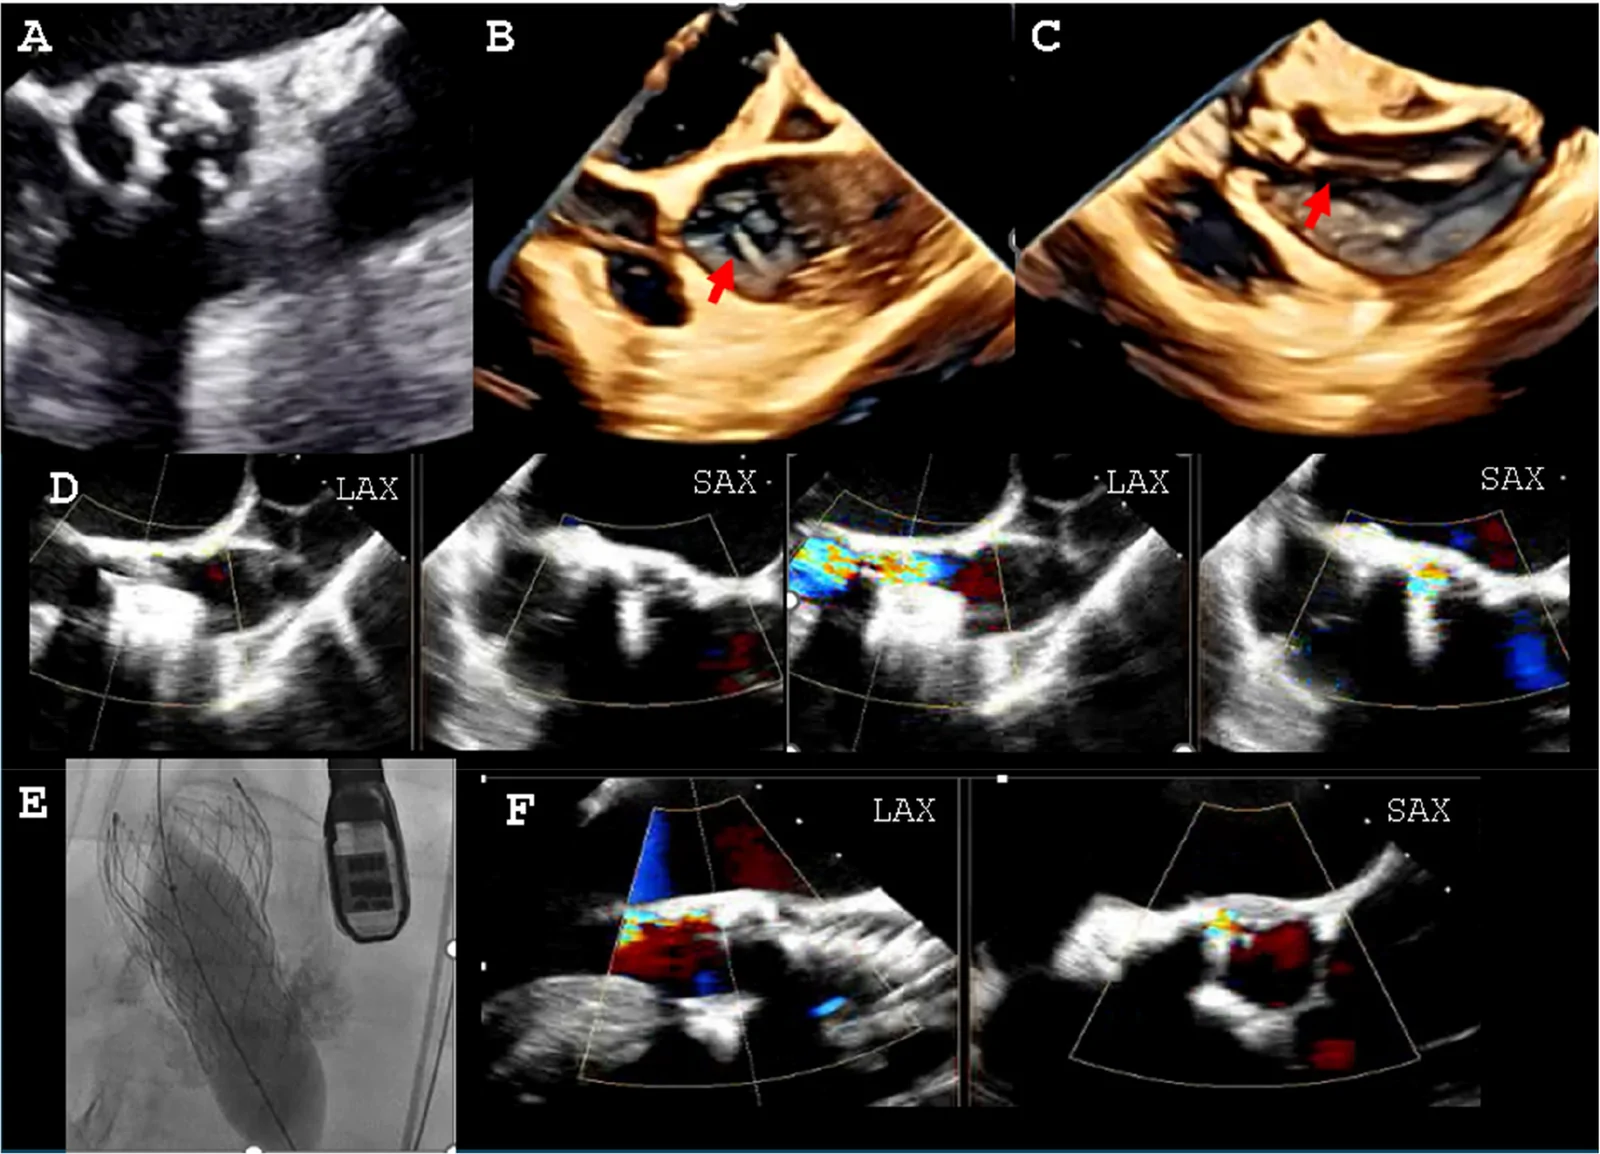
Transcatheter aortic valve implantation (TAVI) in bicuspid aortic valve. A Real-time guidance using mini-transesophageal echocardiography (TEE) is highly valuable in challenging valve anatomy such as bicuspid AV with severe calcification. Under direct 3D TEE guidance, using (volume rendered) (B) *en face* and (C) lateral views of the aortic valve (AV), the guidewire (arrow) is directed towards the narrowed valve orifice that helps in visualizing wire crossing (arrow) of the severely calcified bicuspid AV. D A case of underexpanded transcatheter heart valve in a type 0 bicuspid AV with severe paravalvular leak (PVL) post TAVI on adult-TEE imaging, E which requires reballooning under fluoroscopy guidance. F Final residual mild PVL with circularization of the CoreValve (Medtronic) at the annular level. LAX, long axis; SAX, short axis
